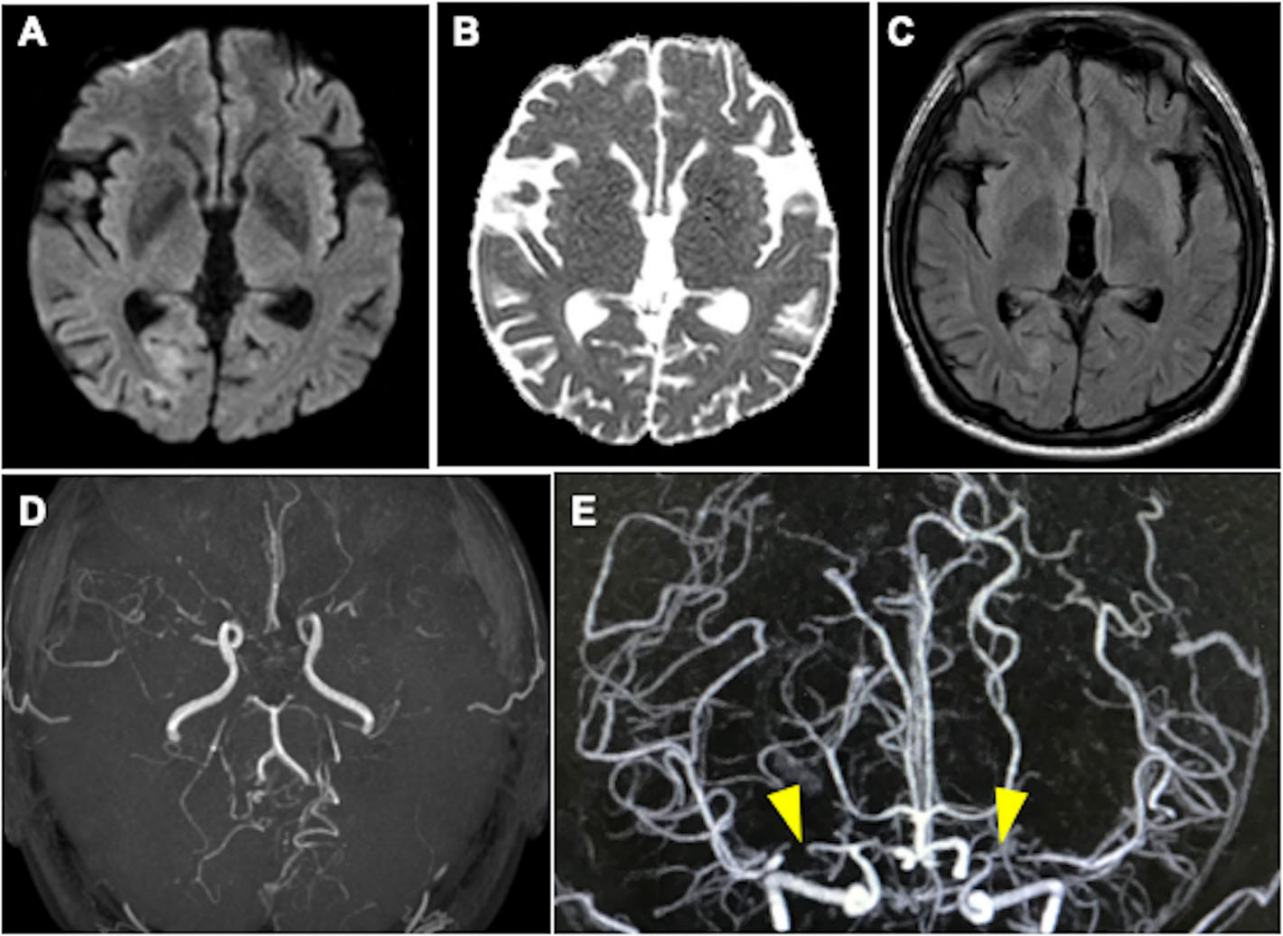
Radiological observations on the first admission a–c. Brain magnetic resonance imaging (MRI) on the first admission shows a hyperintense lesion in the right parieto-occipital lobe on diffusion-weighted imaging (a), apparent diffusion coefficient map (b), and fluid-attenuated inversion recovery (c). d. MR angiography shows stenosis in bilateral middle cerebral arteries (MCAs). e. Three-dimensional contrast-enhanced angiography revealed occlusions in bilateral MCAs (yellow arrowheads)
